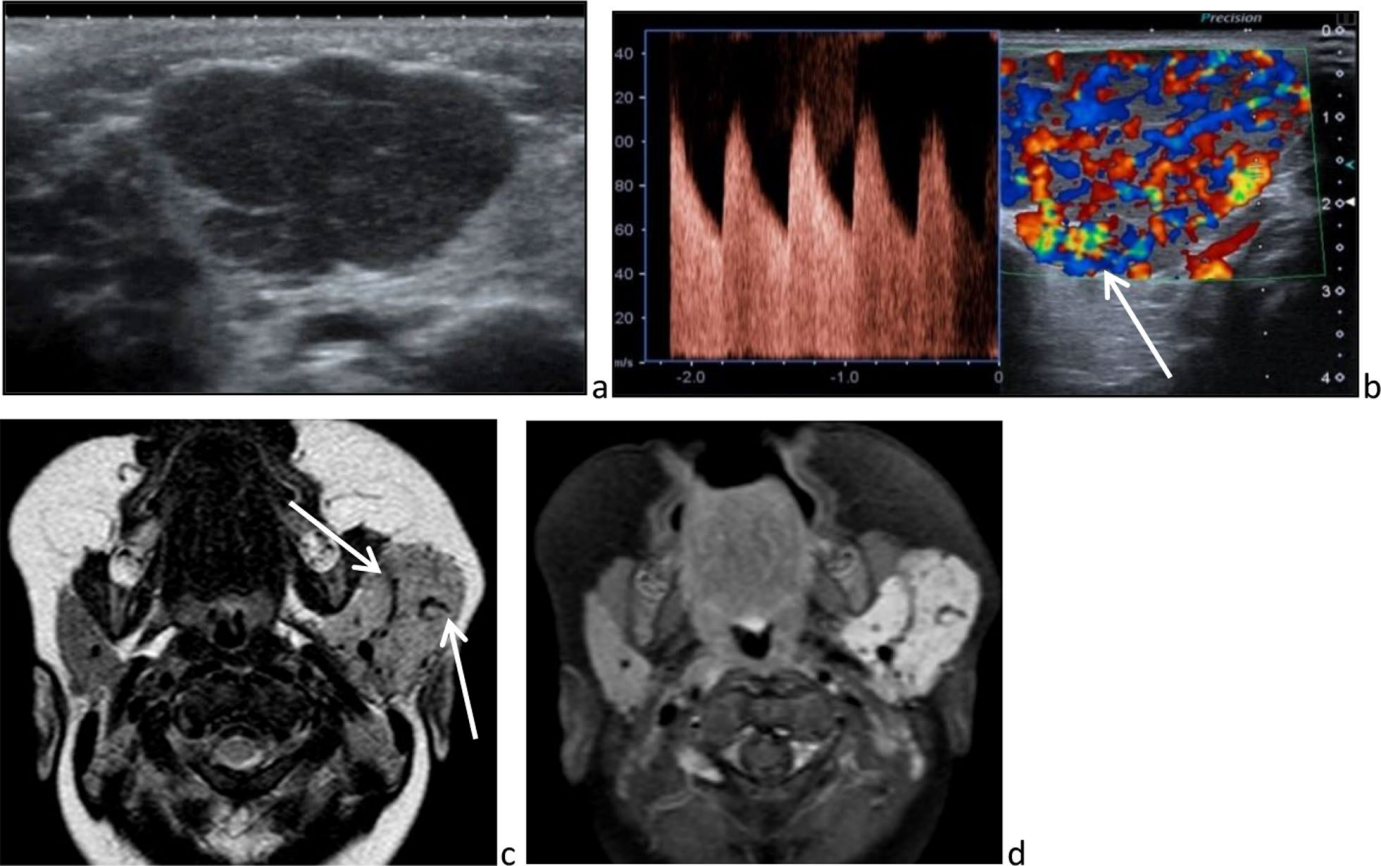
Infantile hemangioma. Lobulated, hypoechoic and hypervascular mass (a) containing high velocity and low resistance arteries (b), on ultrasonography. Diffuse enlargement of the left gland by strongly enhanced mass containing tortuous vessels responsible for flow void images [white arrows], on axial *T*_2_-weighted image (c) and T_1_-weighted image, after intravenous injection of gadolinium chelate (d)
